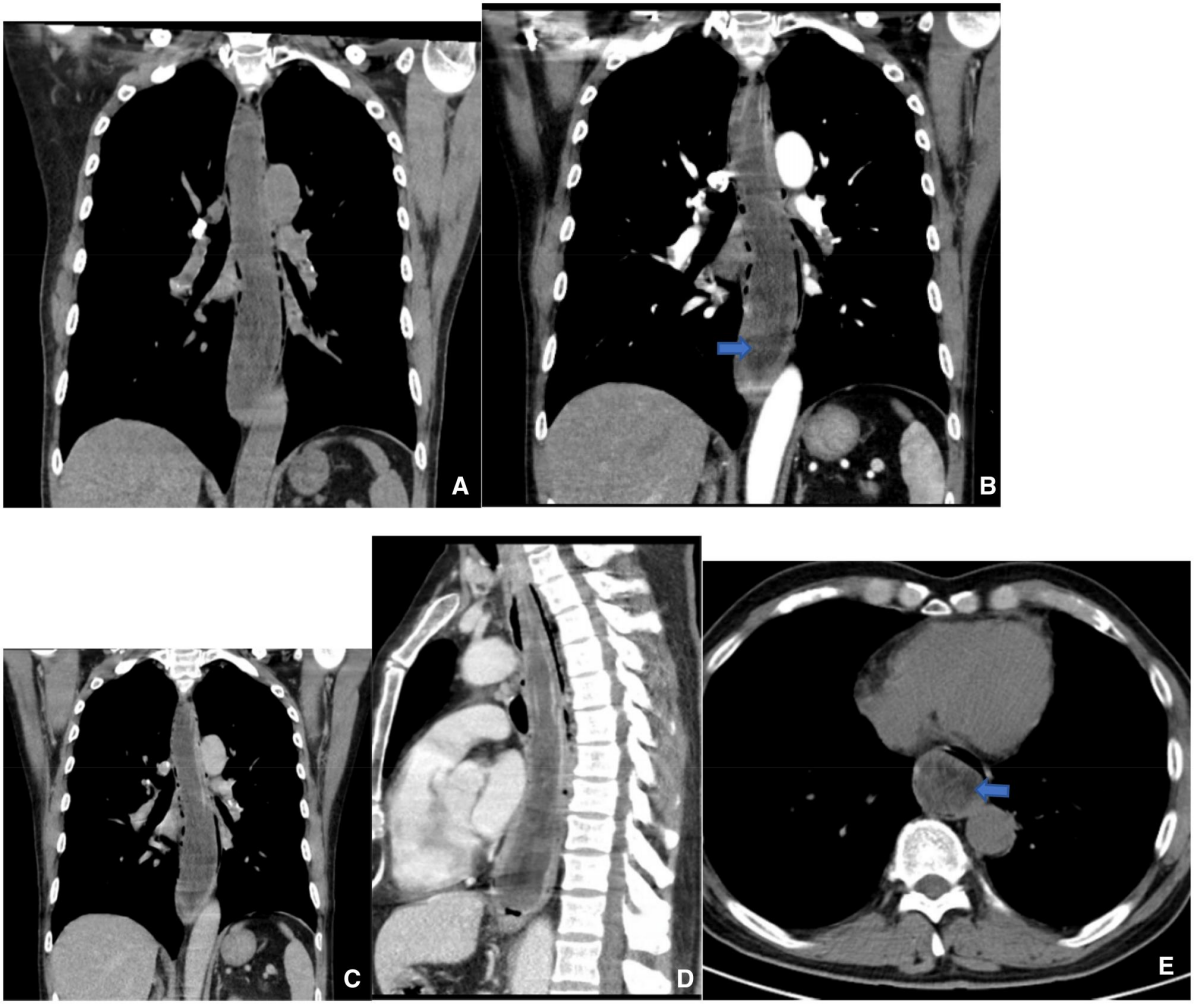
Male, 62 years old, liposarcoma. CT coronal (A, B, C) and sagittal (D) scans show a large mass in the thoracic esophagus (arrowheads), 22 mm long, with a small amount of fat within the lesion (blue arrowheads); CT coronal enhancement scan showing mild enhancement of the solid component.
